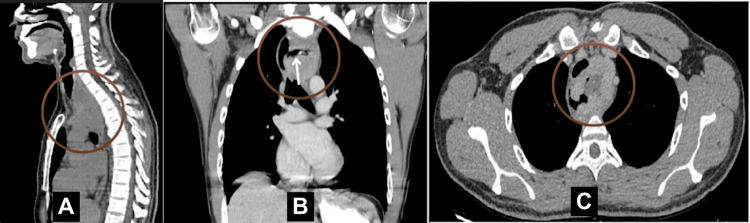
CT chest of the patient’s sagittal (A), frontal (B), and axial (C) views The brown circles in each view show the esophageal schwannoma, while the white arrow in the frontal view (B) denotes the fistulous tract extending from the esophageal lumen to the mass, which was not seen on EGD. EGD: esophagogastroduodenoscopy; CT: computed tomography
